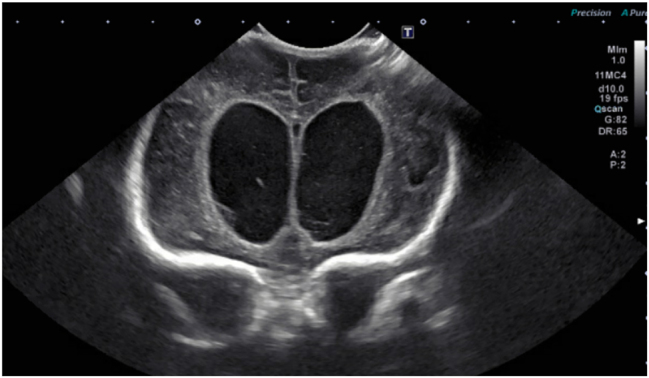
Cranial ultrasound displayed lateral ventricles are enlarged with biparietal diameter measuring approximately 3.9 cm. On the prior exam, this measured 3.6 cm. Intraventricular clot is seen in the bilateral ventricles. There was no significant midline shift. There was an interval evolution of intraventricular clot without extra-axial fluid collection.
